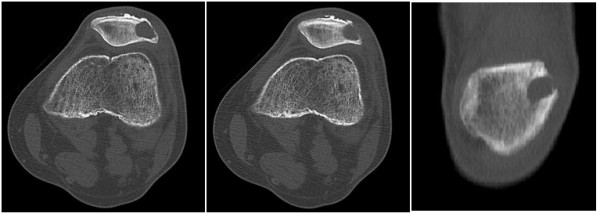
Computed tomography in bone algorithm demonstrates the well defined, low attenuation lesion of the left patella. A breach of the lateral patellar cortex is noted on both the axial and coronal views. No associated soft tissue mass or matrix is identified.
